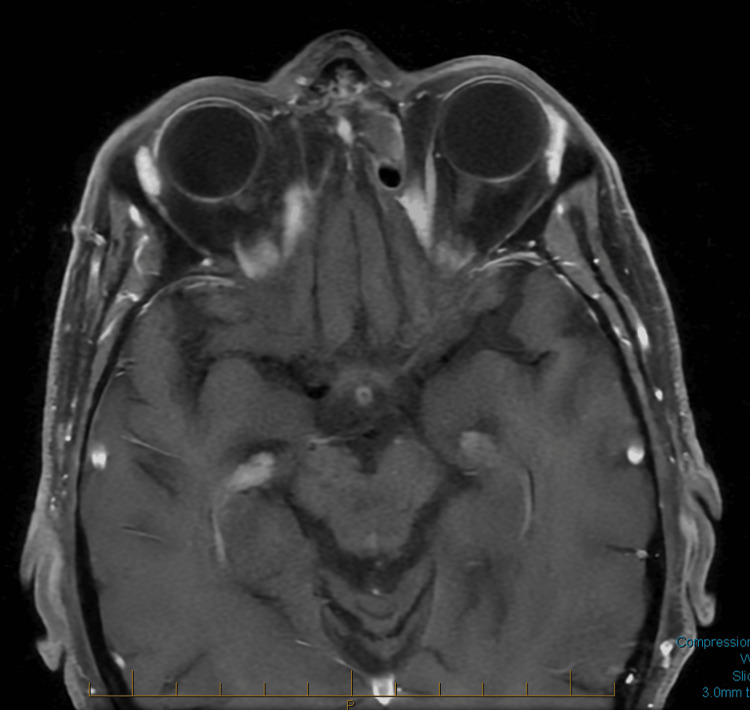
Follow-up MRI orbits (August 20, 2025). MRI: magnetic resonance imaging
